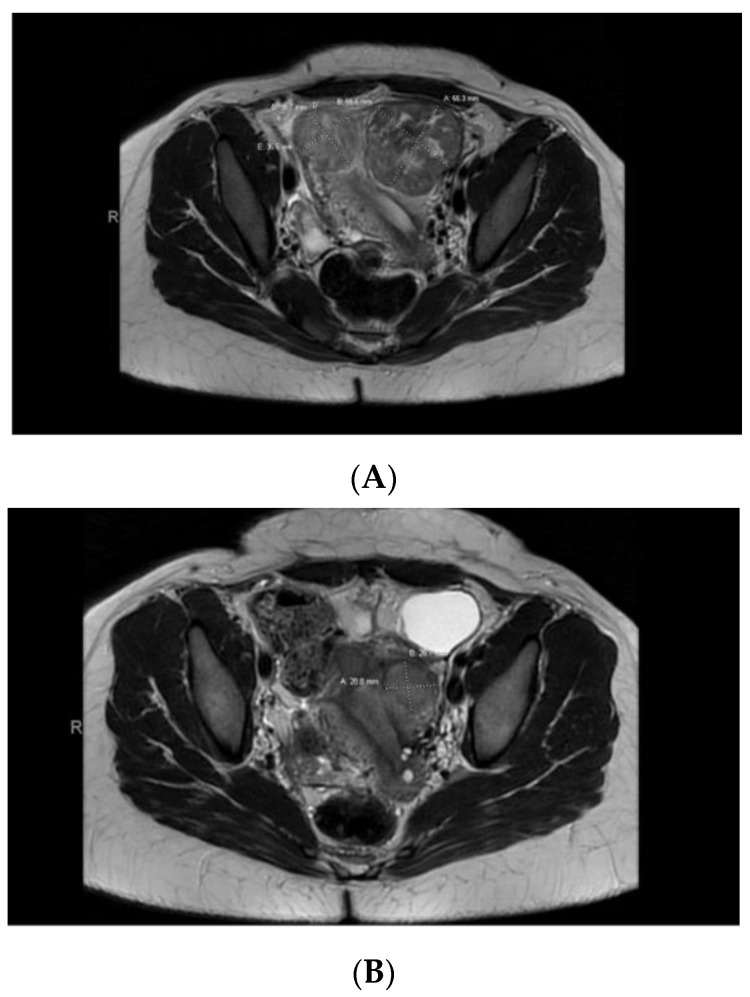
(A) Pelvic MRI pre-myomectomy. (B) Pelvic MRI post-myomectomy.
